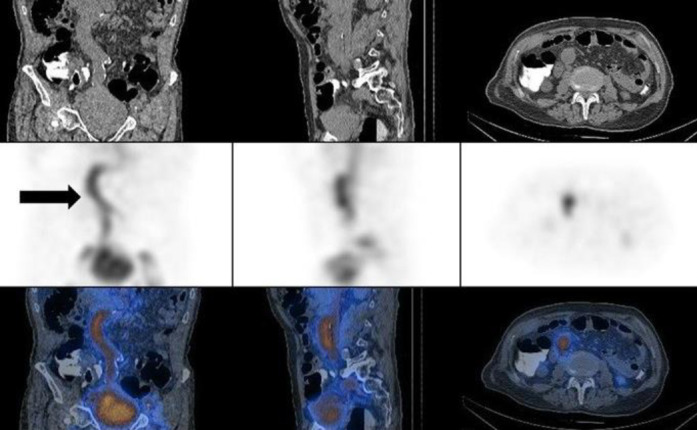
Tortuous inferior vena-cava vein on SPECT/CT images (arrow)
